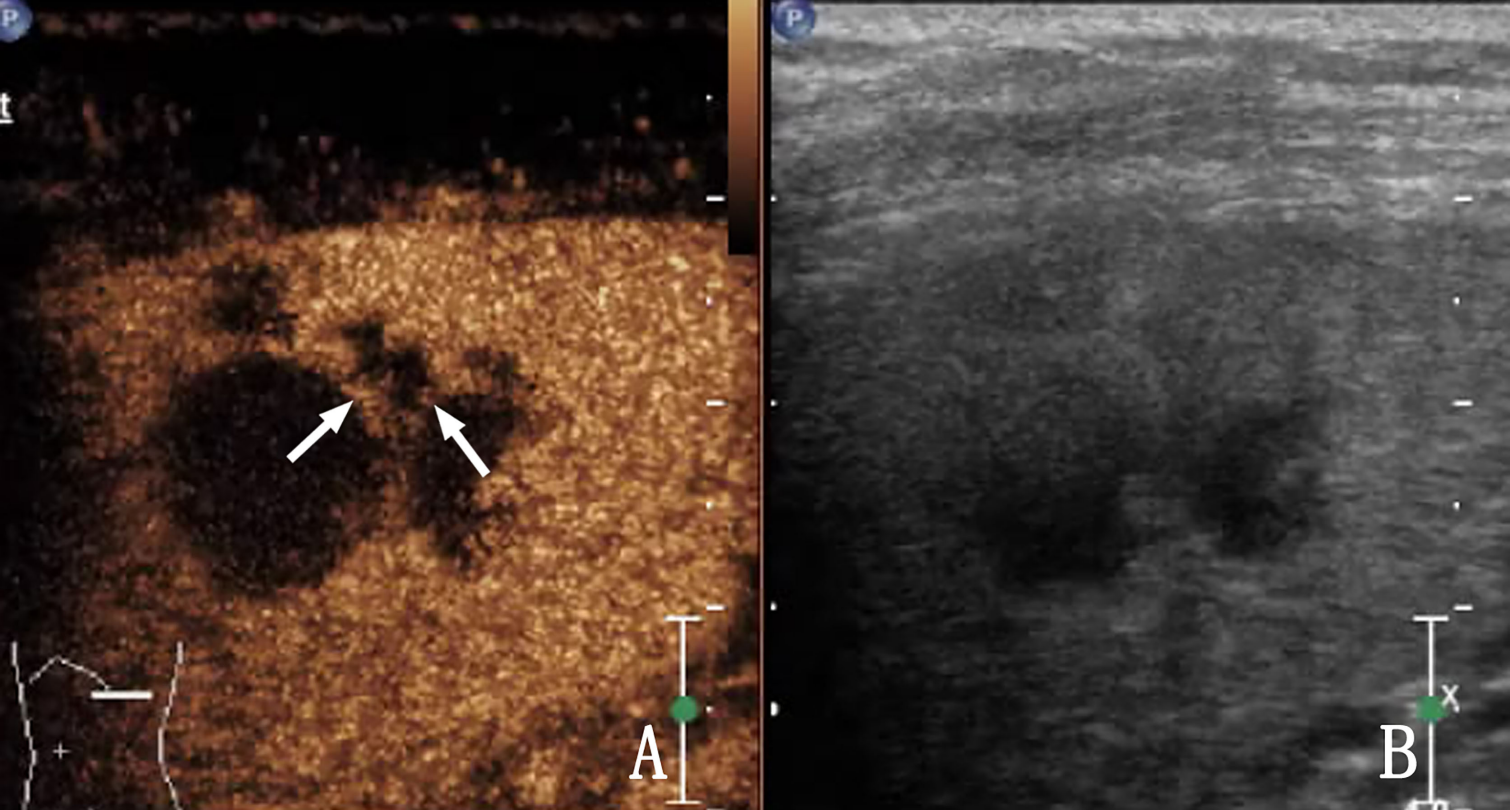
A 28-year-old woman with splenic tuberculosis. (A) Contrast-enhanced ultrasound (CEUS) shows heterogeneous enhancement of the lesion within the spleen, and septal enhancement (arrow) can be observed. (B) Shows a two-dimensional ultrasound (2D US) image.
